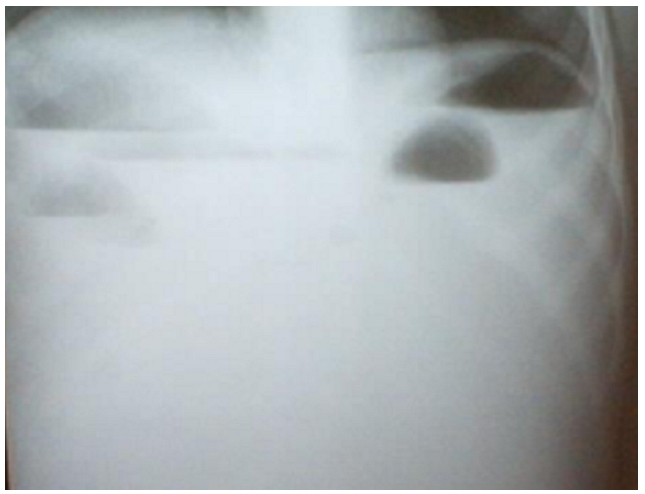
X-ray showing a hydroaeric level driven back to the periphery
